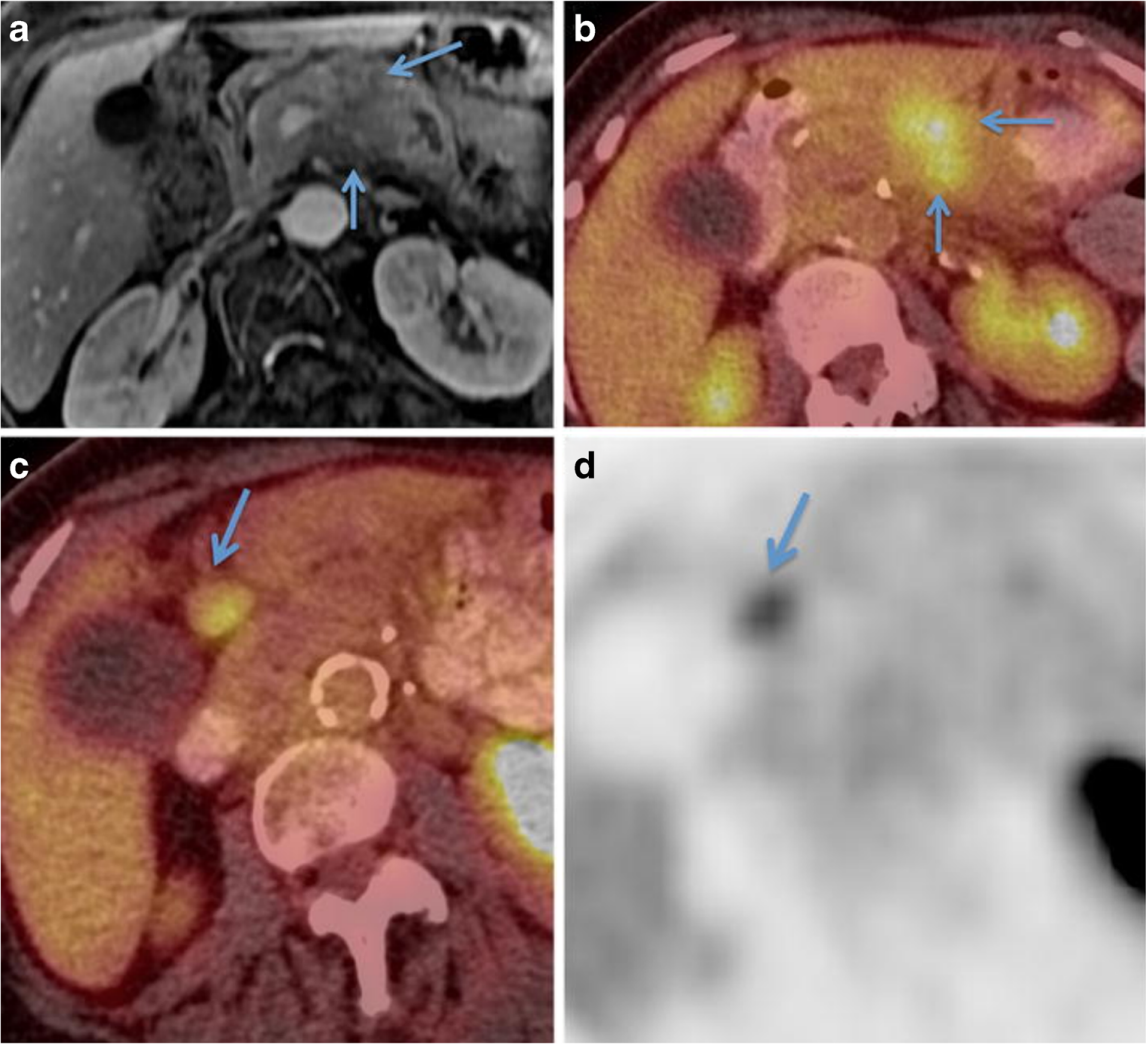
^18^F-FDG PET imaging for initial staging of PDAC in an 80-year-old woman. a The initial staging MR image shows a hypoenhancing mass in the pancreatic body (*arrows*) that has resulted in pancreatic ductal dilatation. b The PET/CT image shows corresponding FDG uptake in the mass (*arrows*). c, d Additionally, the fused PET/CT image (c) and PET-only image (d) show an ^18^F-FDG-avid peripancreatic node (*arrows*). Adapted from Yeh et al. [7]
